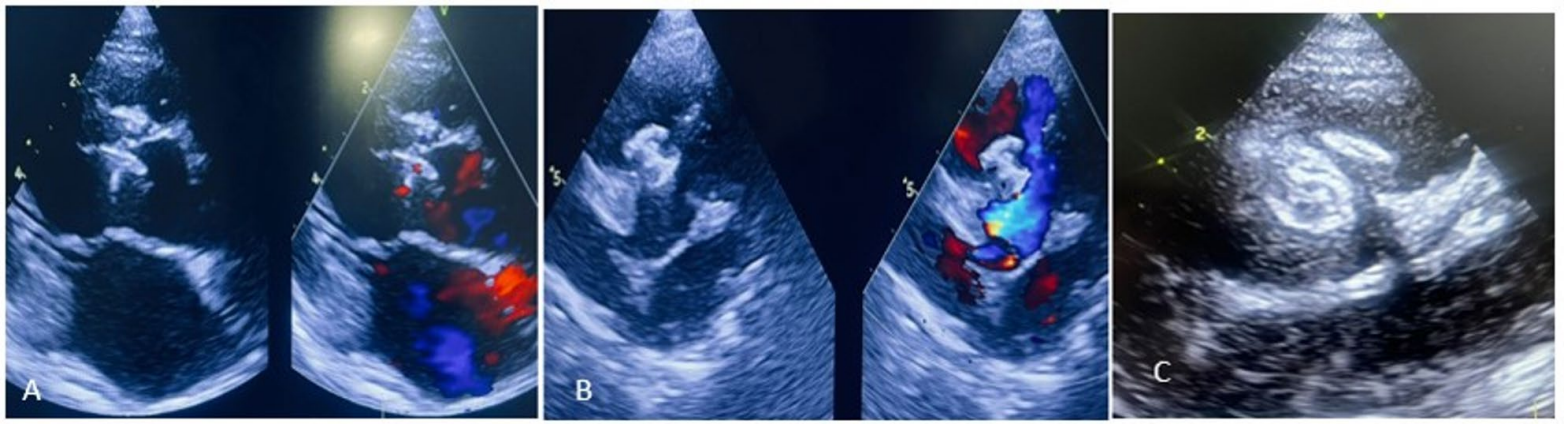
A-C showing the desirable outcomes after device release on echocardiography with perfect positioning of the device and no significant AR
